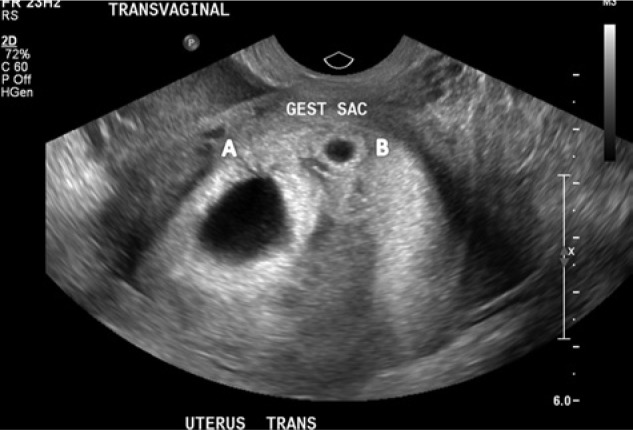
Transvaginal ultrasound of uterus showing 2 asymmetrical twin intrauterine gestational sacs. Fetuses A and B, both with cardiac activity, correspond to 6 weeks 3 days and 5 weeks and 6 days, respectively.
